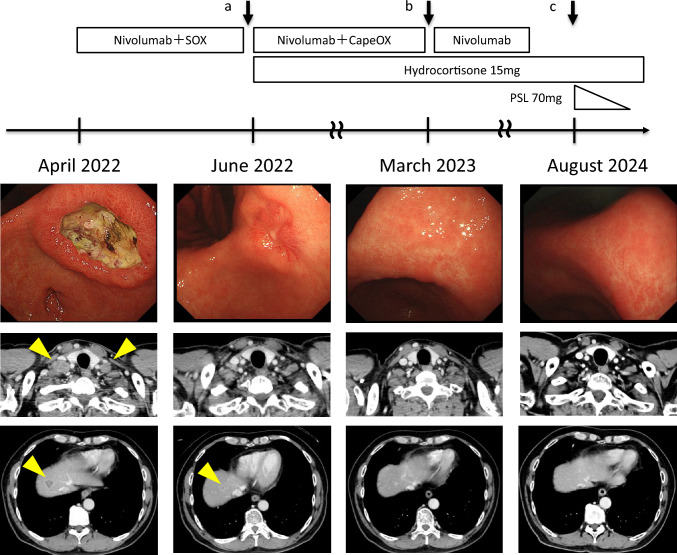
Clinical course with typical endoscopic and computed tomography images. The upper endoscope images show the primary gastric lesion, the middle images show the supraclavicular lymph nodes (yellow arrowhead), and the lower images show the liver metastasis (yellow arrowhead). The series of the three types of images all show the shrinking and disappearance of the lesions. The patient developed hypophysitis with secondary adrenal insufficiency as a grade 3 immune-related adverse event (irAE) at point a, type 1 diabetes (grade 3 irAE) at point b, and colitis (grade 3 irAE) at point c SOX, S-1 (tegafur/gimeracil/oteracil), and oxaliplatin; CapeOX, capecitabine and oxaliplatin; PSL, prednisolone
